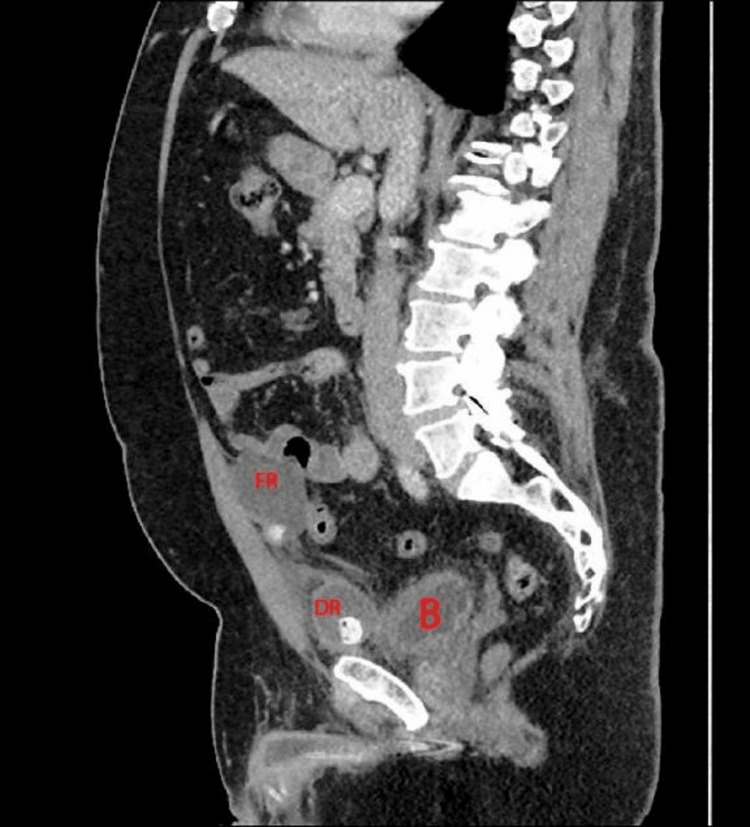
CT urogram showing the DR separated from the bladder The FR is in the submuscular space. B, bladder; DR, decommissioned reservoir; FR, functional reservoir
